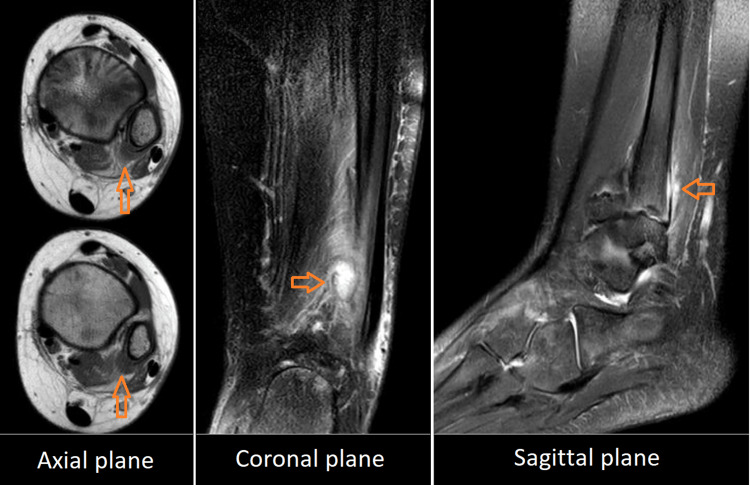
Ankle MRI reveals AHO of the distal fibula complicated by a subperiosteal abscess that has spread to adjacent soft tissue
